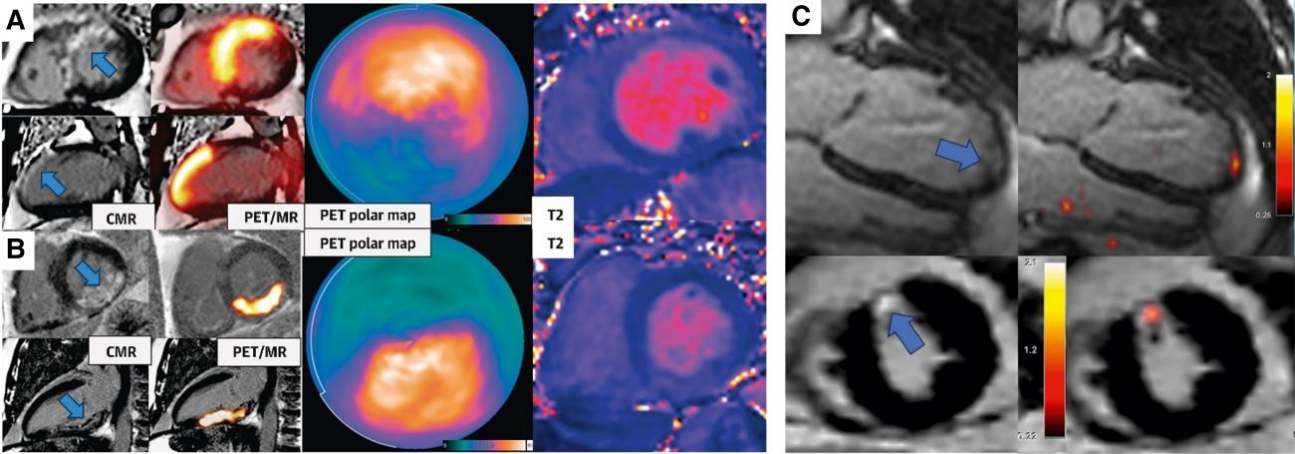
Visualizing acute and chronic fibroblast activity in the infarct and remote myocardium using ^68^Ga-FAPI-46 PET/MRI. Short-axis and 2-chamber PET and LGE-MRI overlay images at *A*) 23 days and *B*) 3 days post-MI. Intense FAPI-46 activity was observed in the infarct zone coinciding with areas of late gadolinium enhancement (blue arrows), and also extending into the peri-infarct regions. *C*) Long-axis and short-axis PET and LGE-MRI images, taken at 2.5 years (top) and 20 years (bottom) post-MI respectively. Low levels of persistent fibroblast activation was detected by FAPI-46 that was confined within areas of chronic infarction. Reproduced from Barton *et al.*, open access under Creative Commons CC-BY 4.0.^34^
